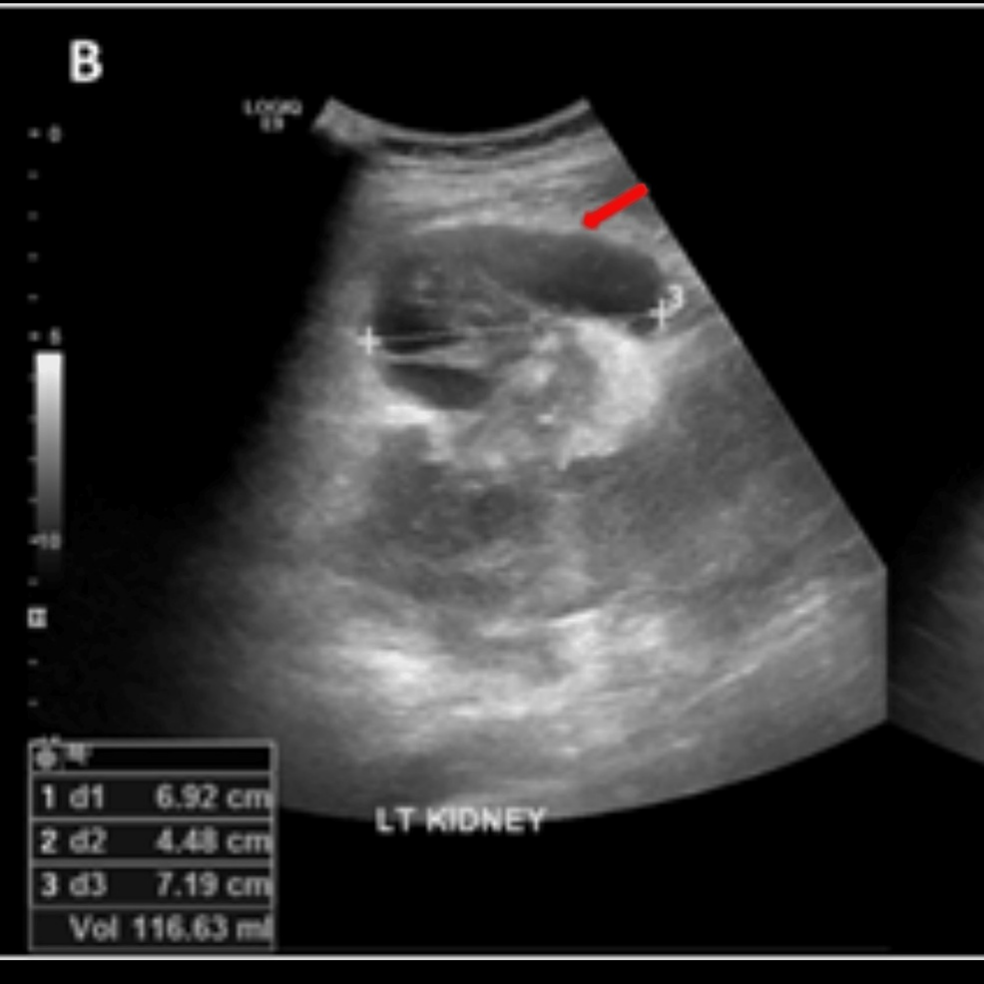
Ultrasound urinary tract showing perinephric hematoma (red arrows).
